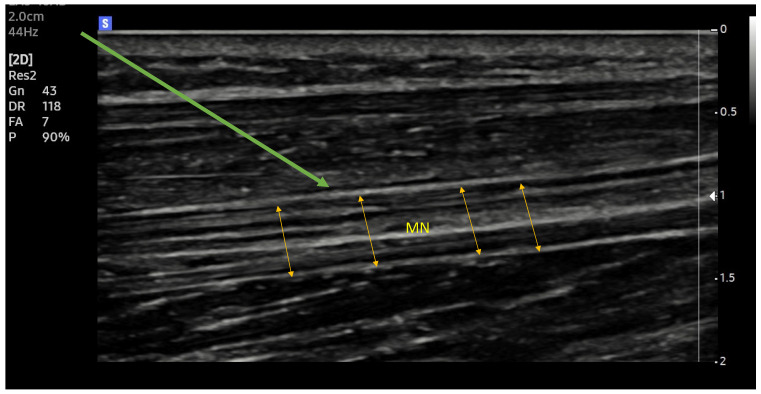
Median nerve. Ultrasound longitudinal (long axes) view. MN (yellow arrows)—median nerve; Green arrow—needle trajectory; Arrowhead—extraneuronal needle placement.
